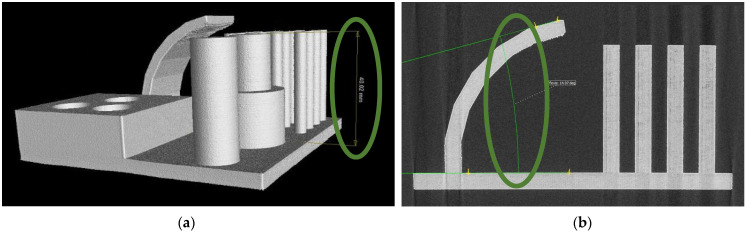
Dimensions check on CT. (a) Pin height (Plasty Mladeč); (b) overhang angle measurement (Gembird).
